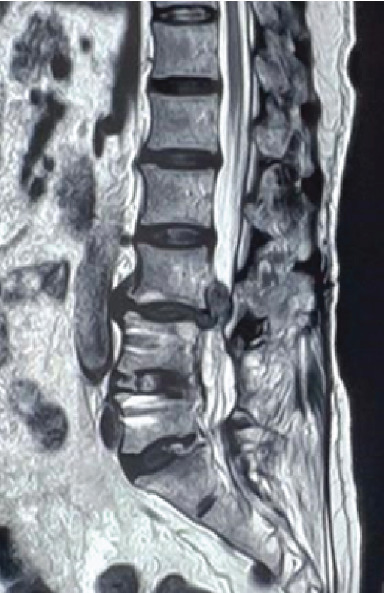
Lumbar MRI shows L3-4 adjacent segment degeneration and lumbar disc herniation with upward migration.
